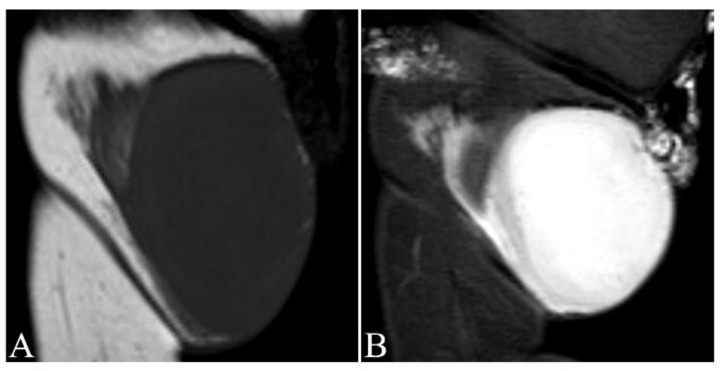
Magnetic resonance imaging (MRI) of dermatofibrosarcoma protuberans on the left thigh of an 8-month-old girl. (A) MR T1-weighted image exhibiting well-defined homogeneous isointense lesion; (B) T2-weighted image showing a well-defined mass with intermediate-to-marked homogeneous hyperintensity with infiltration of the adjacent fat plane and encasement of the gracilis muscle at the level of this image.
